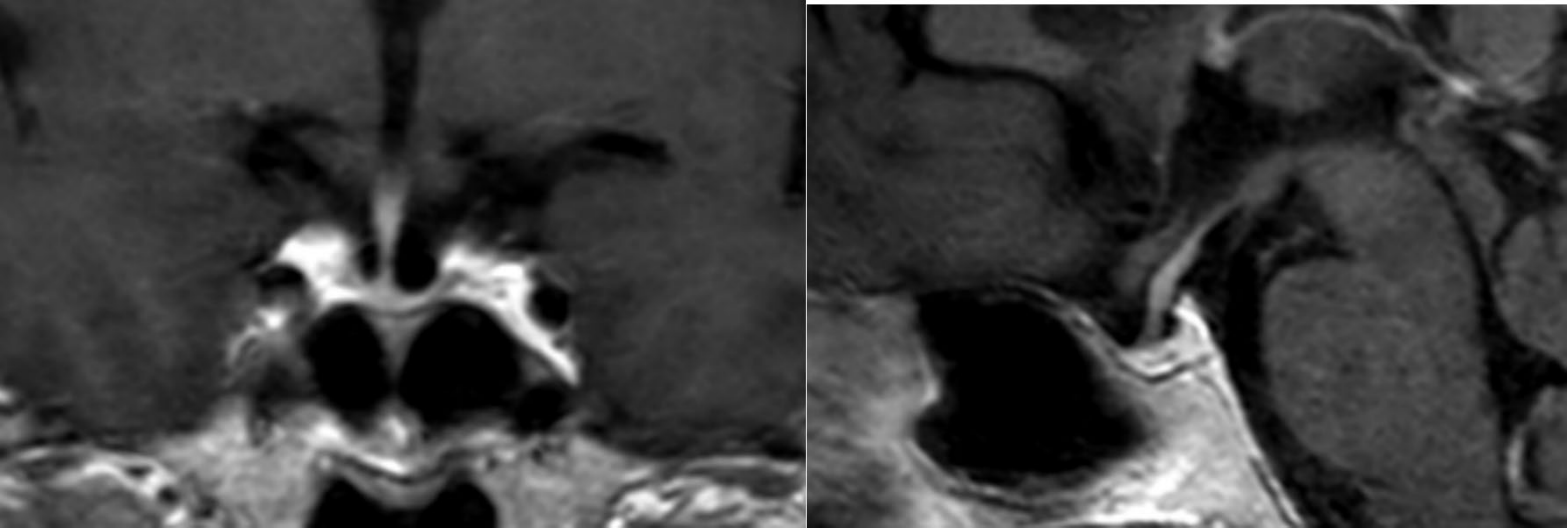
Pituitary MRI of the case showing empty sella appearence
